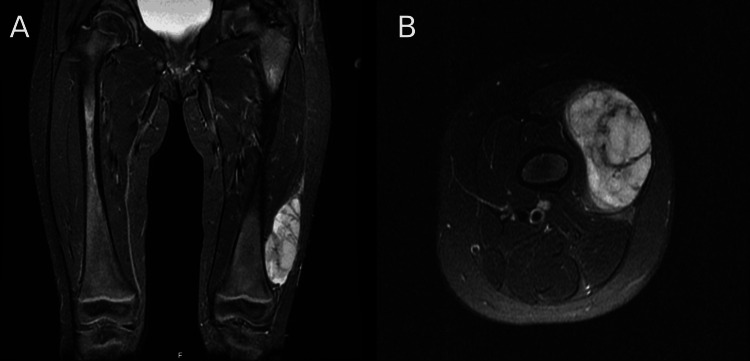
A. Coronal view of MRI shows a well-defined hyper-intense soft tissue mass with marked enhancement at the lateral lower left thigh. B. Axial view of MRI shows no evidence of local bone invasion
